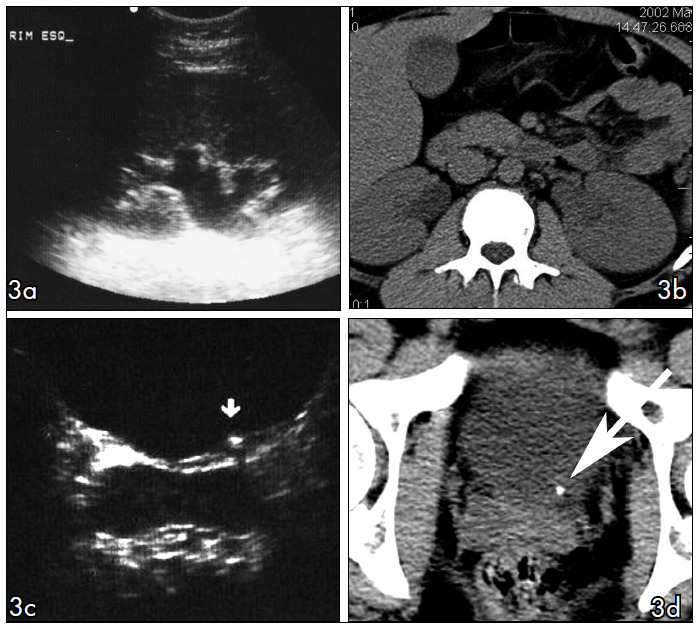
Comparison between ultrasound (US) and computed tomography (NCT) in two different patients. (a) Coronal identification of the left kidney showing collecting system dilatation, which was confirmed in NCT (b). US scan at bladder level showing stone at left ureterovesical junction (arrow in c), confirmed by NCT (d).
